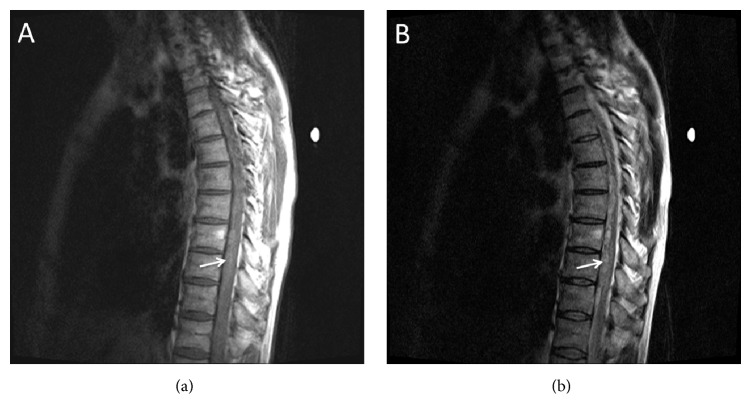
Preoperative MRI sagittal views T1 (a) and T2 (b) of the thoracic spine. White arrow indicates subdural hematoma.
